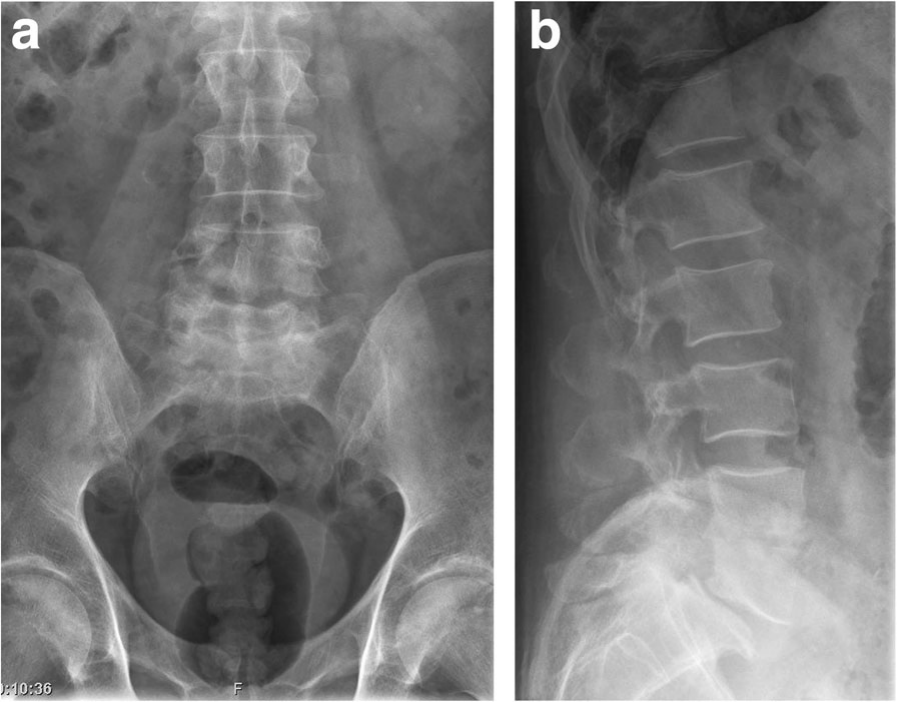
a and b Preoperative radiograph of the lumbar spine anterior and lateral on 6 Jan 2016: narrowing of the intervertebral space L4 and L5 with osseous destruction of base plate L4 and upper plate L5
